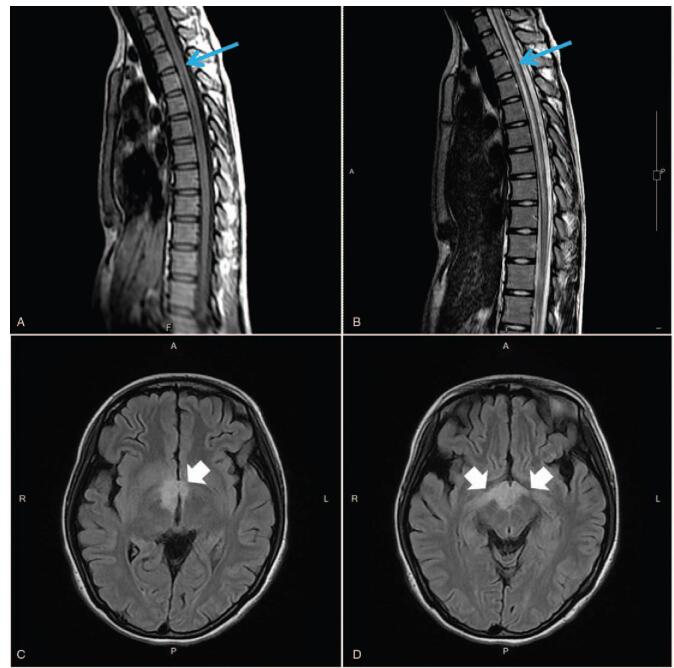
Spinal MRI findings (arrows) indicating transverse myelitis. A, T1 sagittal. B, T2 sagittal with high signal through spinal canal at C6-T9 level. Brain MRI findings (arrows). C, T2 axial with hyperintensity in hypothalamic region. D, T2 axial with hyperintensity in hypothalamic region and bilateral post-chiastic optic tract.
